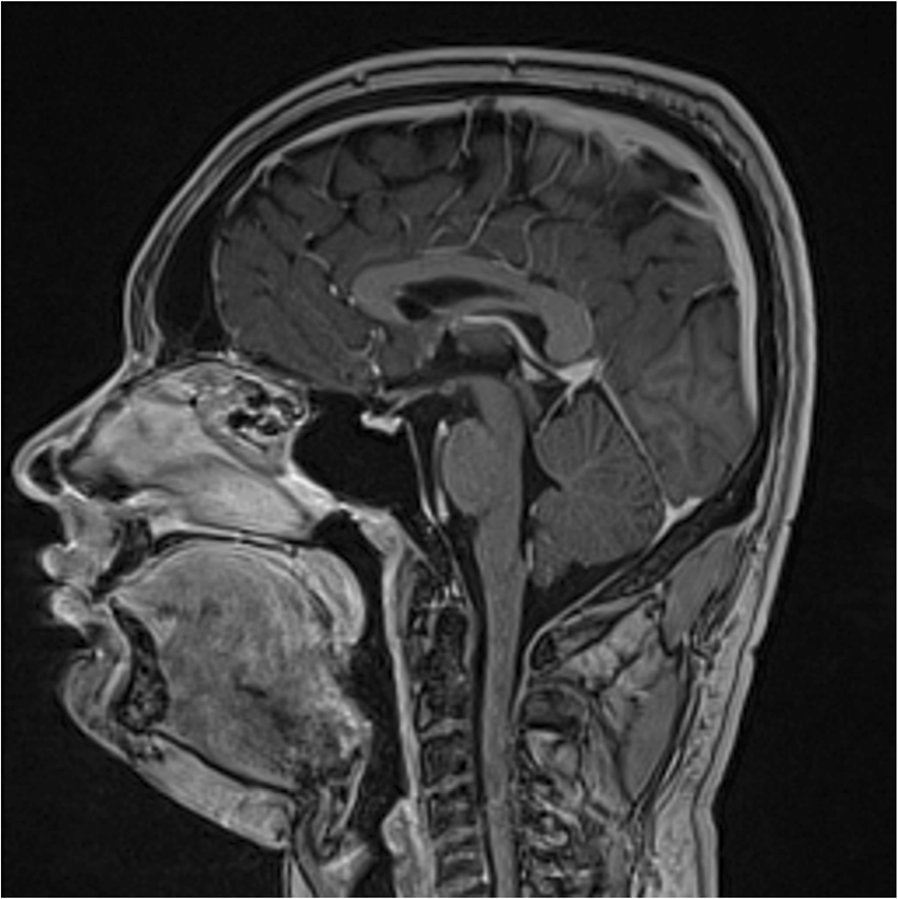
Magnetic resonance image of the brain (sagittal view) showing the absence of a hypothalamic lesion
